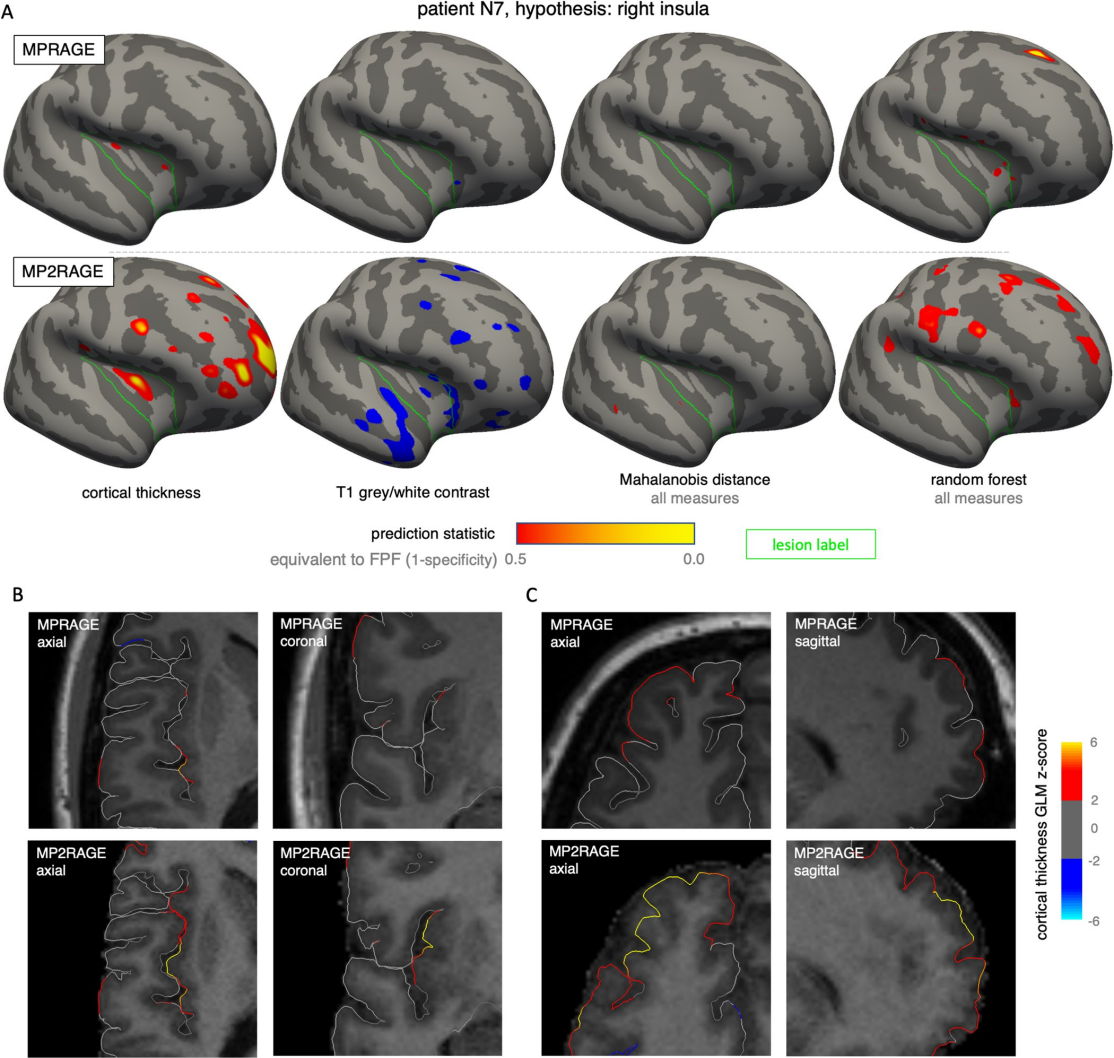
Exemplary lesion detection results in an MRI-negative patient. (A) Overlays of the prediction statistic on the inflated template surface for different selected univariate and multivariate approaches. The colorscale is aligned according to the false-positive fraction in each case. The green outline depicts the border of the lobar hypothesis label. (B, C) Original image data with overlaid tracings of the reconstructed pial surface, colored according to the cortical thickness GLM z-score, showing the maximum value in the temporal hypothesis label (B) and frontopolar (C). Overall, the results agree well with the clinical constellation in this patient: Whereas the hypothesis was formally defined as right insular, upon intracranial EEG also seizures originating from right frontal were recorded; in the end, the multifocal localization contraindicated surgery.
